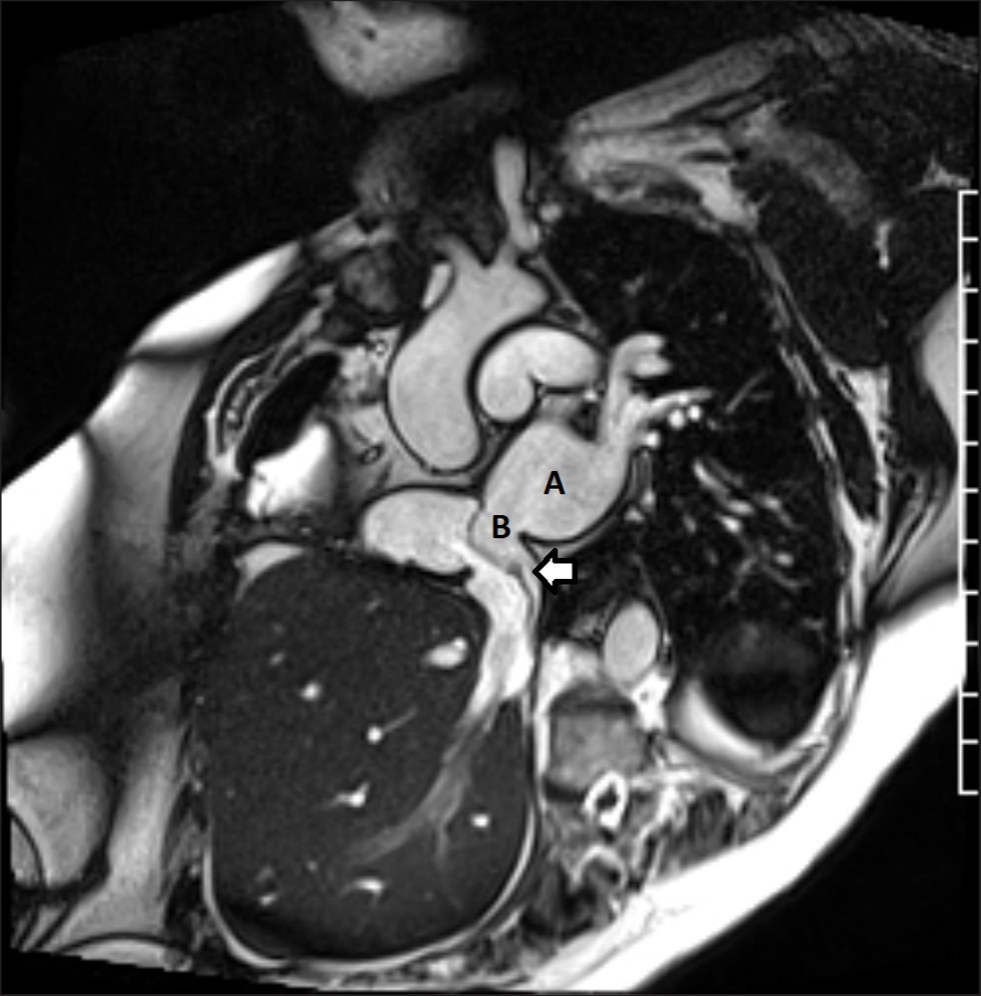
Cardiac magnetic resonance imaging post scimitar repair showing the scimitar vein baffle draining into the left atrium through a surgically created atrial septal defect (ASD). (A) Left atrium; (B) surgical ASD. Arrow: scimitar vein baffle
